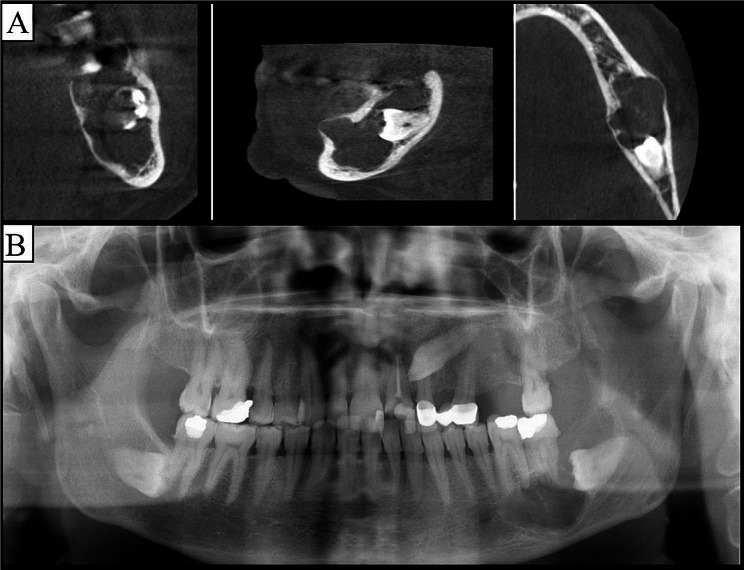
(A) CBCT image of a DC in the left mandibular region, with scalloped borders and partly well-defined margins in coronal, sagittal and axial planes, (B) Panoramic image of the same patient
